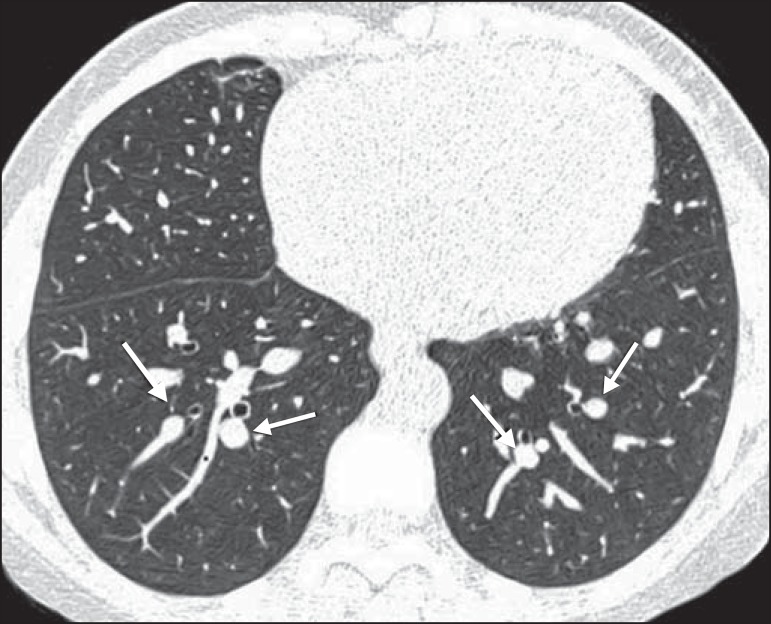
Axial chest CT scan showing increased caliber of the peripheral pulmonary
